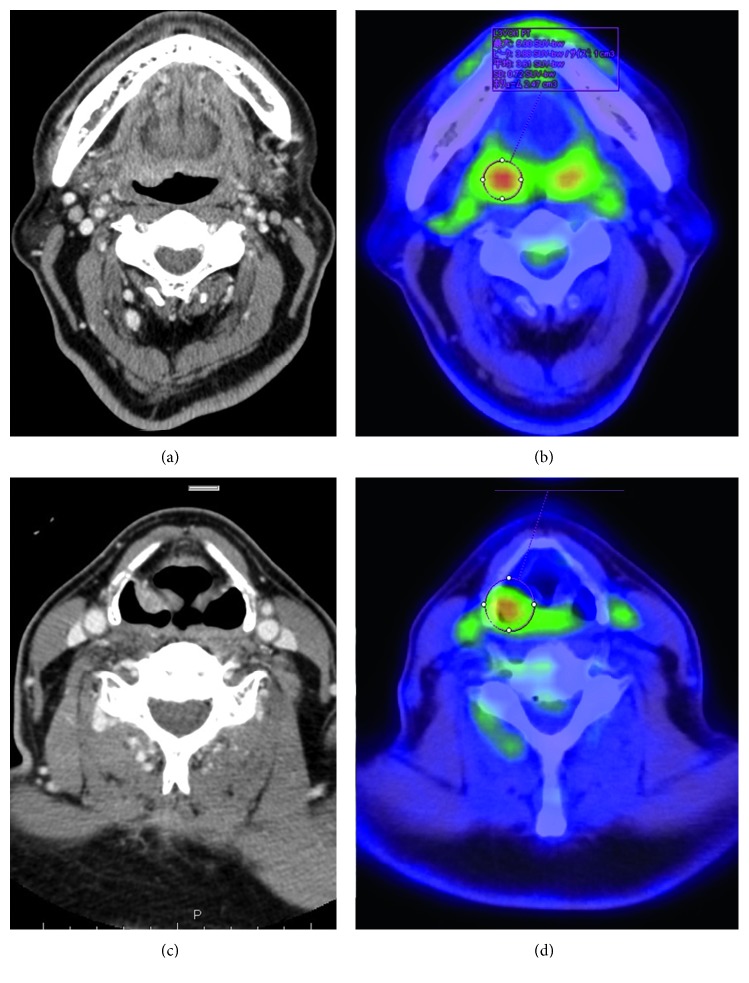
Contrast-enhanced CT and FDG PET/CT. Thickening of the pharyngeal wall with irregular contrast enhancement is observed on the right side of the tongue base and in the right piriform recess. SUVmax is 2.0 on the right side of the tongue base and 4.2 in the right piriform recess.
